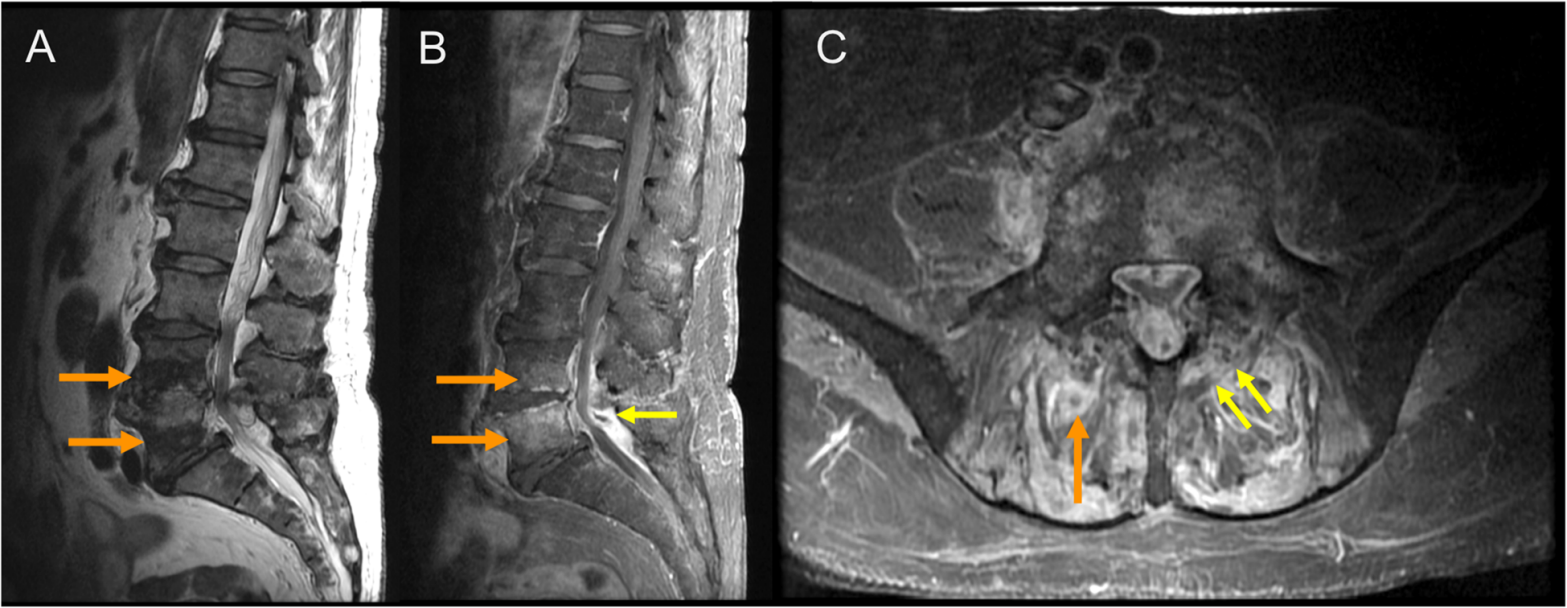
a Sagittal T2-weighted turbo spin-echo pulse-sequence shows remarkable low signal intensity in the caudal endplates of L4 and L5 (arrows), as well as the interspinous area. b Sagittal T1-weighted spin-echo pulse-sequence with fat sat obtained after administration of intravenous gadolinium reveals pathological contrast enhancement of the caudal endplate of L4 and cranial endplate of L5 (arrows), with sparing of the intervertebral disk L4-L5. Moreover, there is striking enhancement of the intraspinal epidural space at the level of L4-L5 with a small epidural abscess at L5 (small arrow). c Axial T1-weighted spin-echo pulse-sequence with fat sat obtained after administration of intravenous gadolinium shows a second small abscess in the right erector spinae muscle (arrow). Note the involvement of the posterior elements, most prominent on the left side (small arrows)
